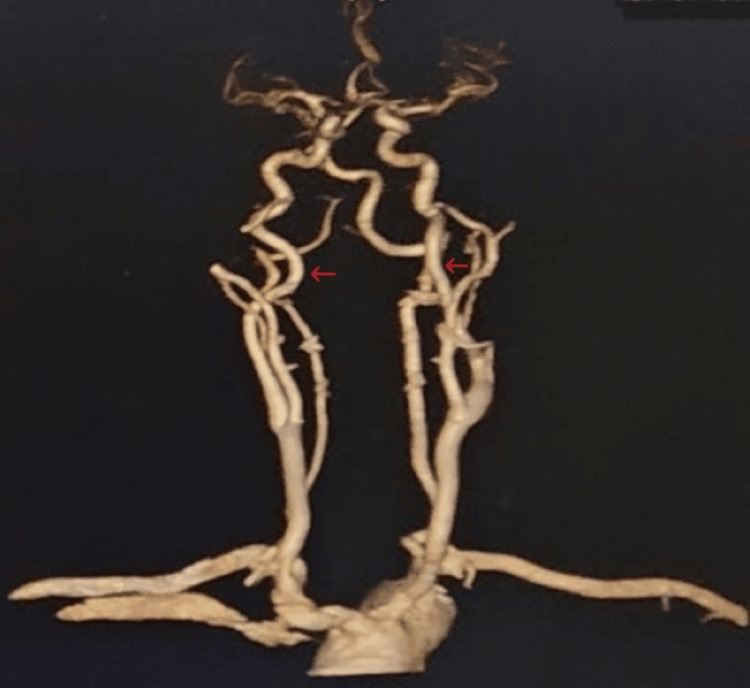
Three-dimensional reconstruction of head and neck CTA. No evidence of internal carotid artery stenosis was found. CTA: computed tomography angiogram.
